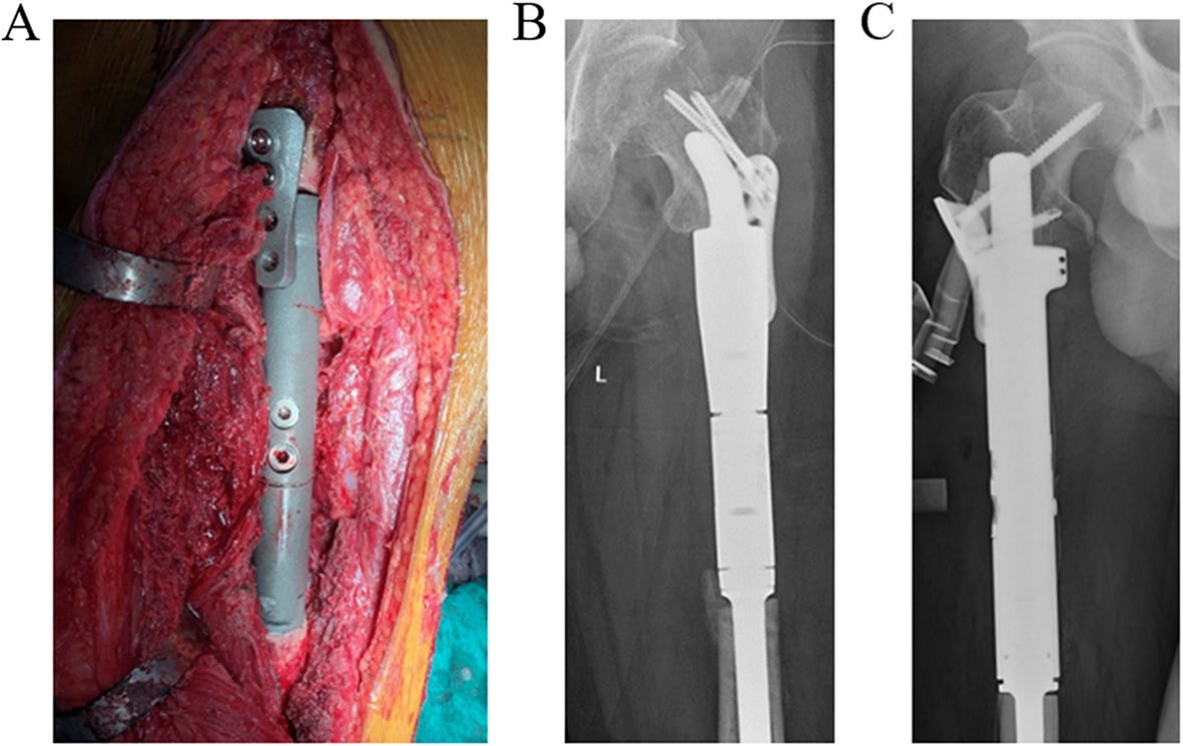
The successful placement of 3D-printed femoral prostheses. A The intraoperative implanted 3D-printed prosthesis of Patient 3. B The postoperative immediate X-ray of the left femur in Patient 1. C The postoperative immediate X-ray of the right femur in Patient 3
